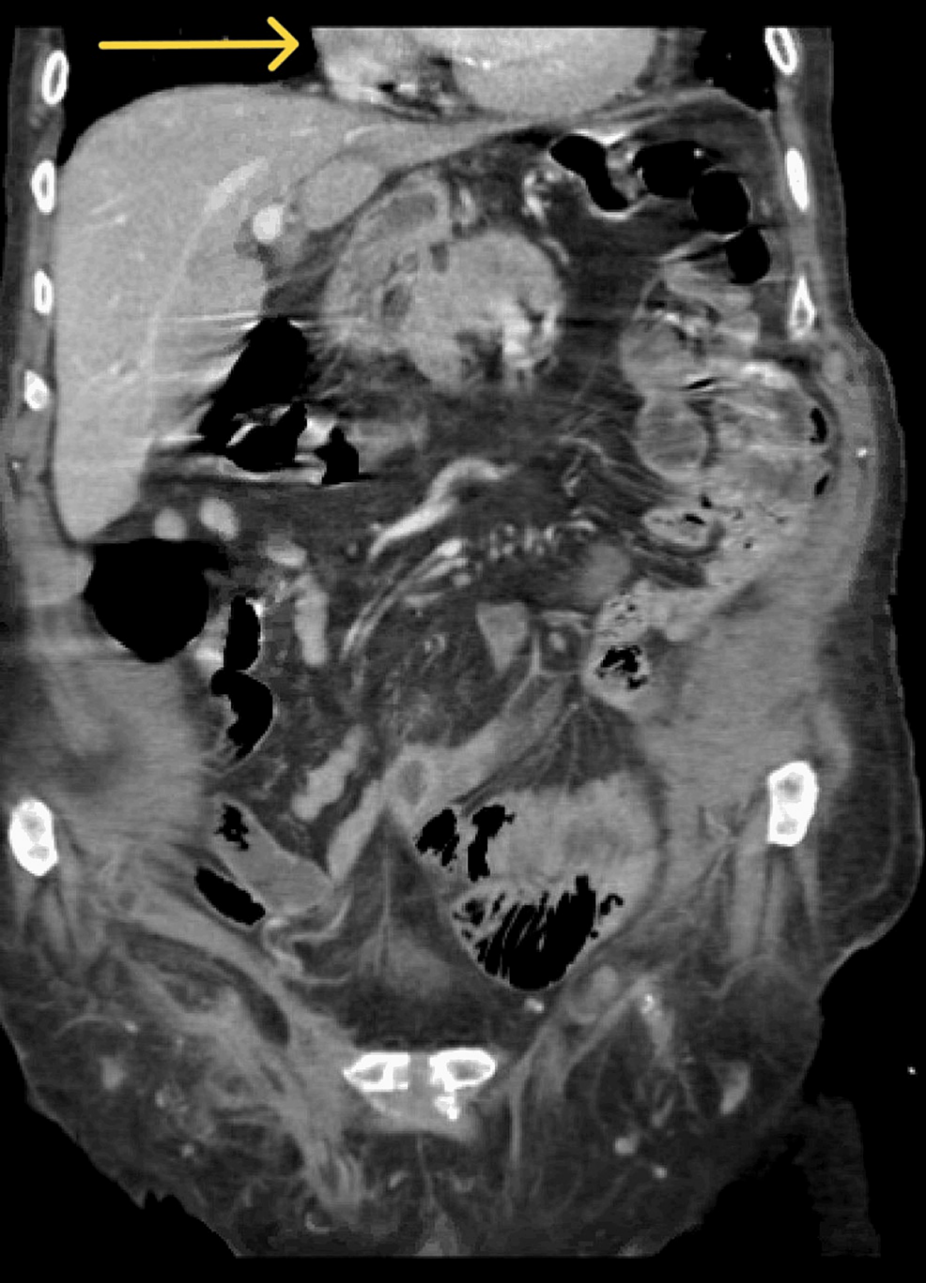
Abdominal CT with contrast (coronal view) The image shows another view of a large hiatal hernia containing the entire stomach and part of the duodenum (arrow) in the chest cavity.
